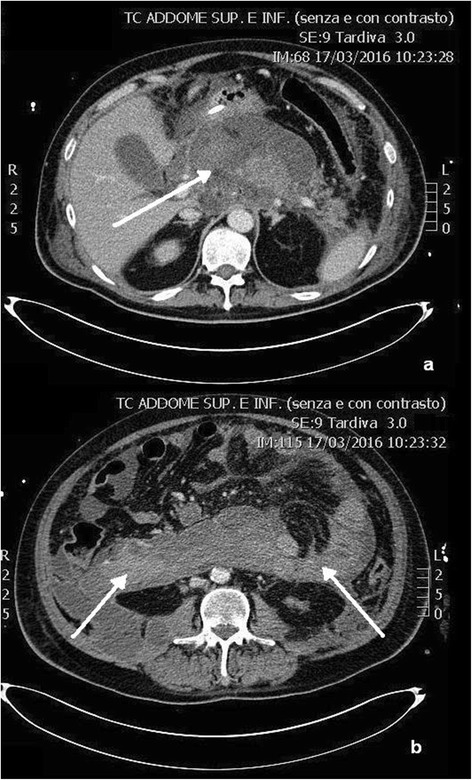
CT scans after 4 weeks. Acute pancreatic necrosis is replaced by a central walled-off pancreatic necrosis (a, *arrow*) and lateral retroperitoneal fluid collections (b, *arrows*)
